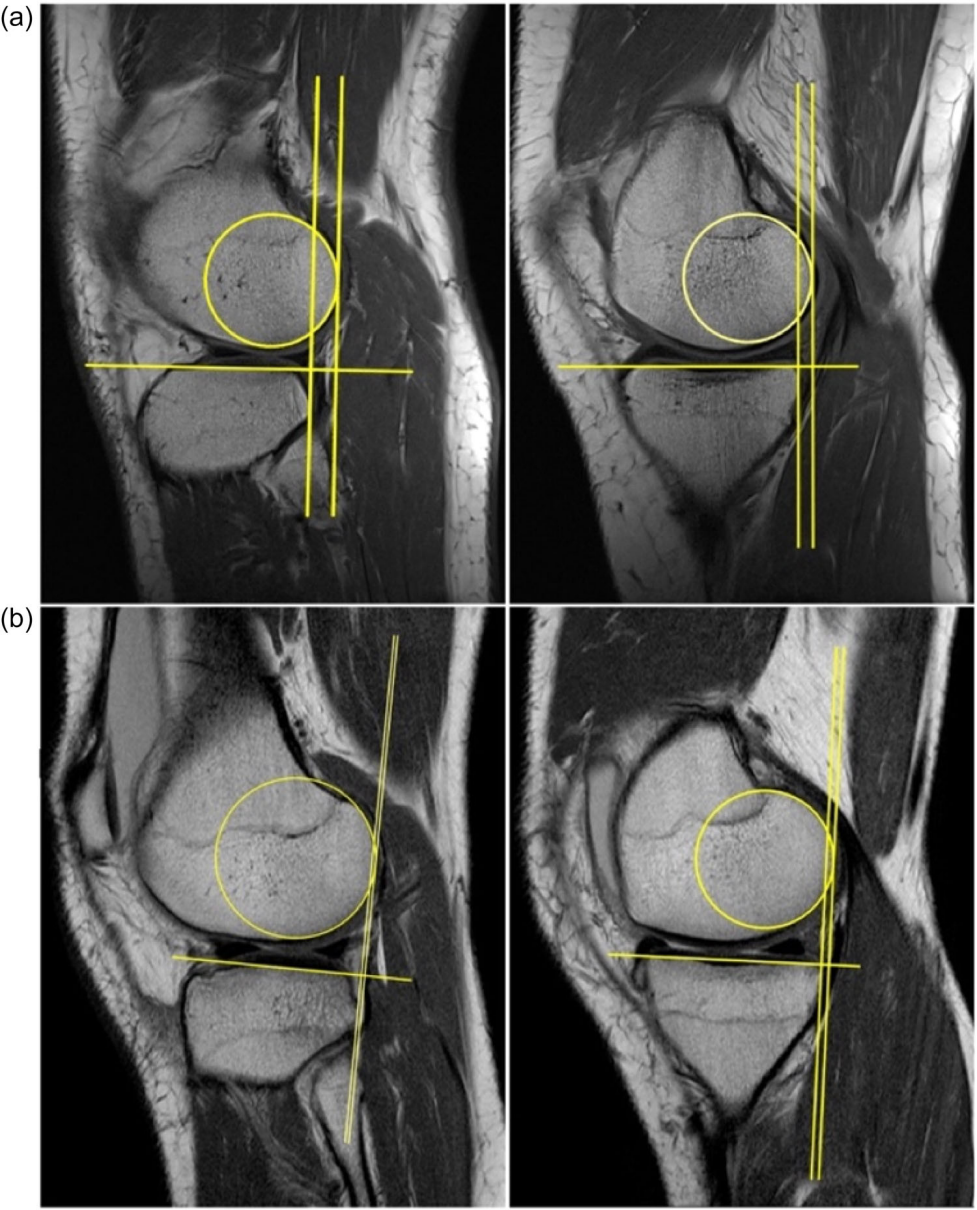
MRI subluxation measurements. Static anterior subluxation of the lateral (left) and medial (right) tibial plateau in patients with an i‐ACL and high (a) and patients with c‐ACL‐I and a low (b) rotatory knee laxity [27]. Preoperative sagittal T1‐weighted MRI scans of the patients' knees in the supine position were used to measure static tibial subluxation for all three cohorts in the MC and LC as previously described [27, 45]. The decisive factor for identifying the sectional image plane for the MC was the fermoral insertion of the medial gastrocnemius tendon and the most medial incision of the fibula in the tibiofibular joint for the LC. First, a best‐fit circle was placed over the subchondral portion of the posterior femoral condyle. Next, a straight line was drawn from the most anterior to the most posterior part of the tibial plateau and extended beyond the tibia. Another straight line was then drawn perpendicular to the previously line and tangentially approximated to the posterior edge of the best‐fit circle. Finally, a third straight line, also perpendicular to the tibial plateau, was drawn and approximated to the posterior side of the tibia. The resulting distance between the two parallel straight lines was defined as the degree of static tibial subluxation respectively translation. ACL, anterior cruciate ligament; i‐ACL, intact ACL; c‐ACL‐I, complete ACL injury; LC, lateral compartment; MC, medial compartment; MRI, magnetic resonance imaging.
